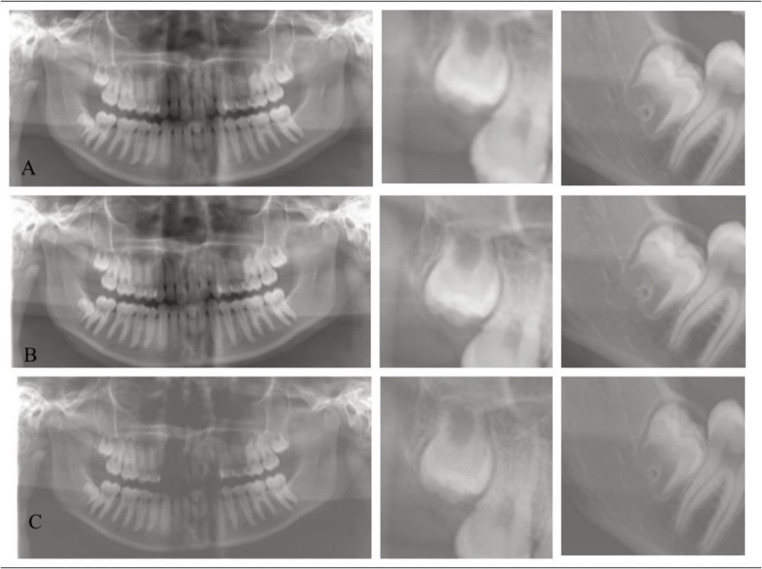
Radiographs and cropped images obtained with the three acquisition protocols. A: 70 kVp, 12.5 mA, 3393 µGy; B: 66 kVp, 8 mA, 1841 µGy; C: 66 kVp, 3.2 mA, 720 µGy
